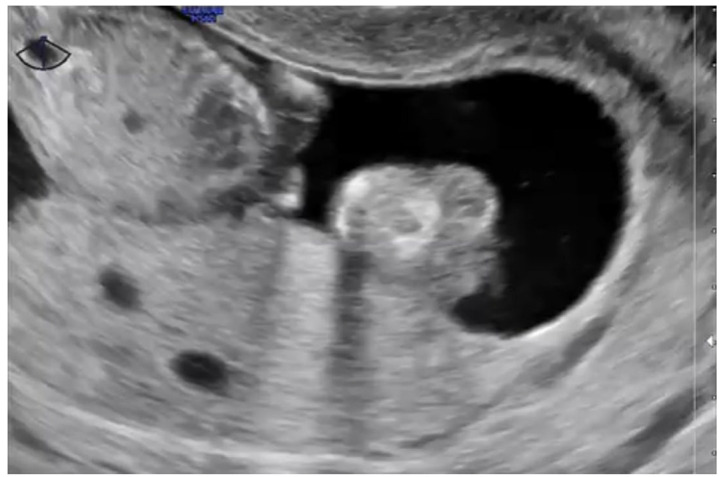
Ultrasound image of a partial hydatidiform mole. The scan reveals a non-viable fetus of an approximate gestational age of 15 weeks. The placental region shows two large anechoic regions consistent with cystic changes. The images refer to clinical cases managed by the authors.
